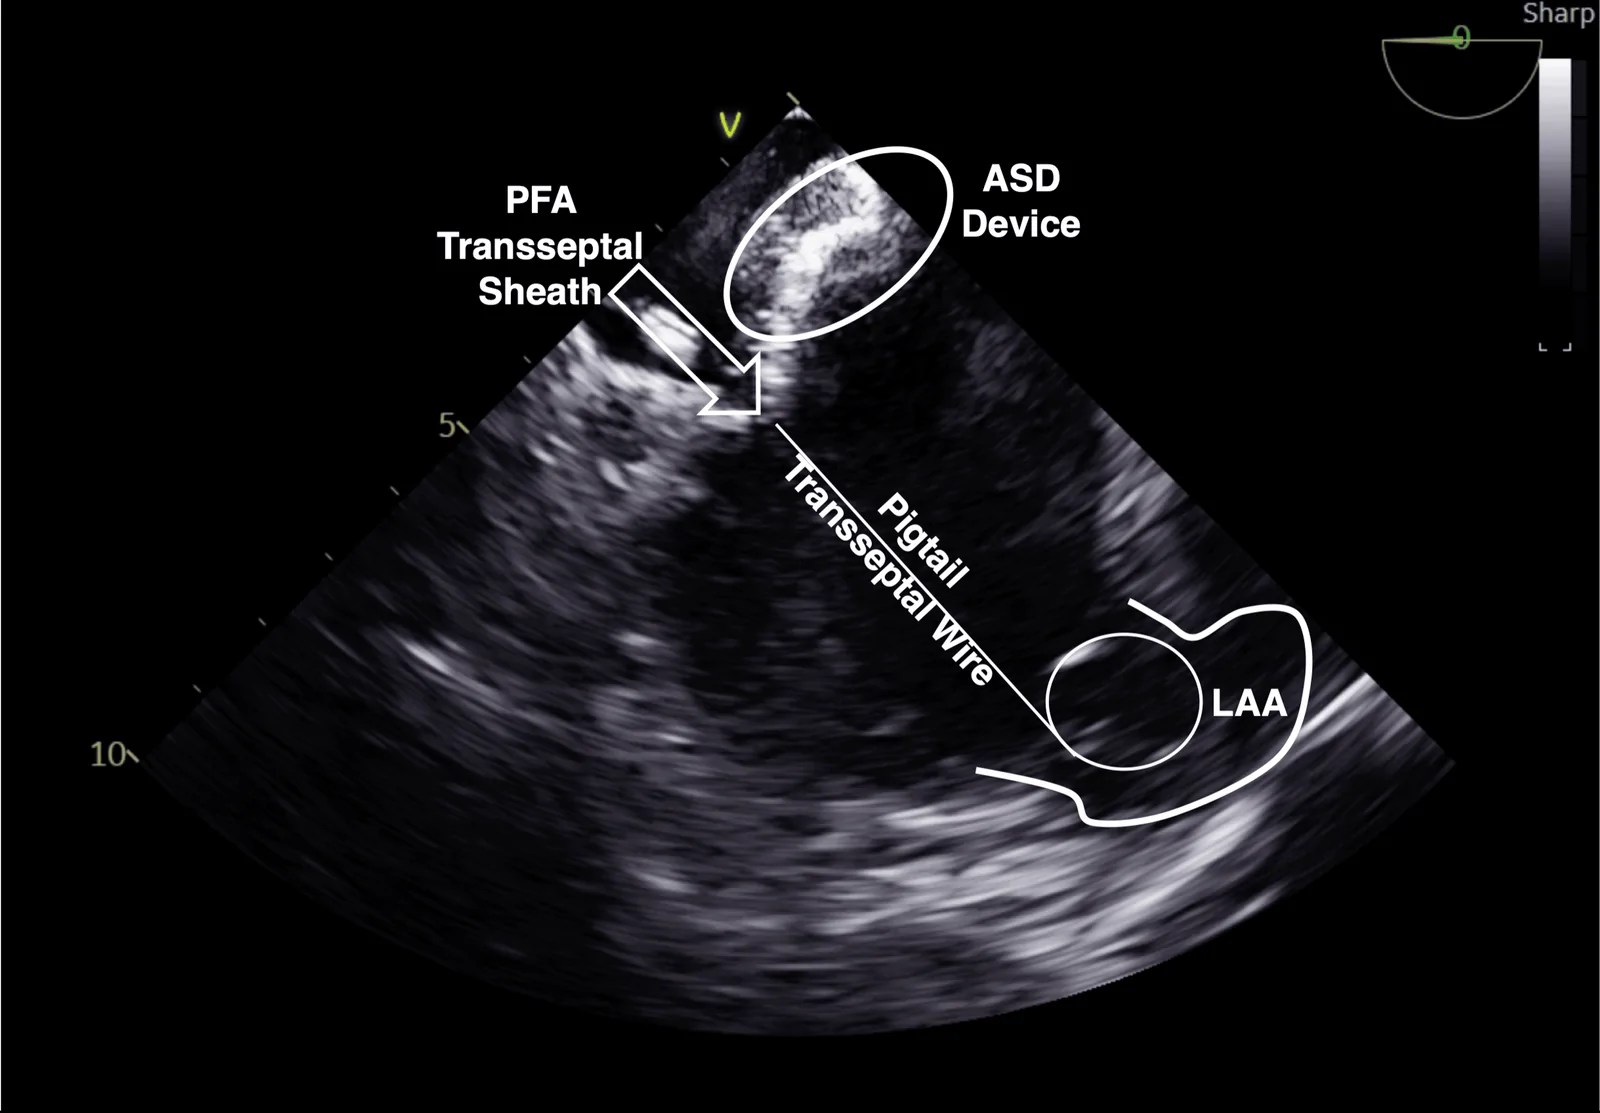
Pre-transseptal puncture intracardiac echocardiography Pre-transseptal puncture intracardiac echocardiography demonstrating an inferior transseptal trajectory directed toward the left atrial appendage, inferior to the ASD closure device. ASD: atrial septal defect; PFA: pulsed field ablation; LA: left atrium; LAA: left atrial appendage.
